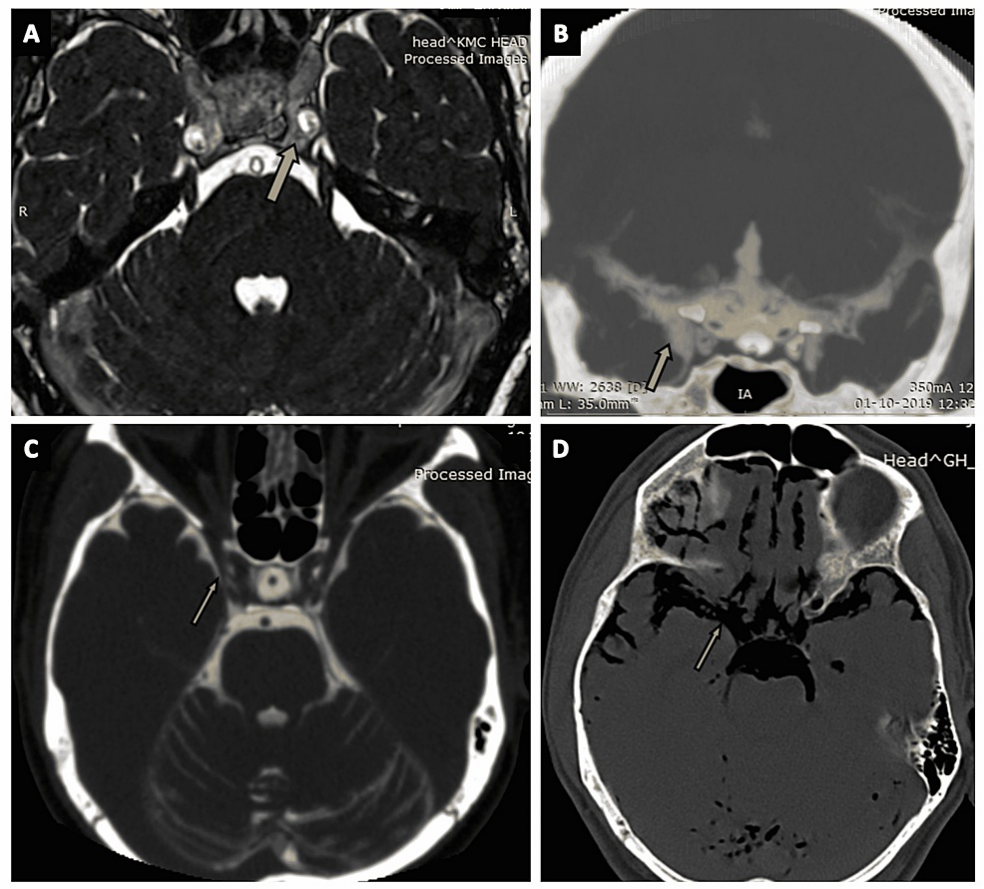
The carotid cistern. (A) The carotid cistern (arrow) located medial to the temporal lobe as seen on an axial section of the routine three-dimensional (3D) constructive interference in steady state (CISS) MRI sequence. (B) The carotid cistern (arrow) visualized on a coronal section of CT cisternography in a case of suspected CSF rhinorrhea. (C) The carotid cistern (arrow) as seen on an axial section of CT cisternography in a case of suspected CSF rhinorrhea. (D) The carotid cistern (arrow) as seen on an axial section of CT brain (negative contrast) in a case of pneumocephalus.
